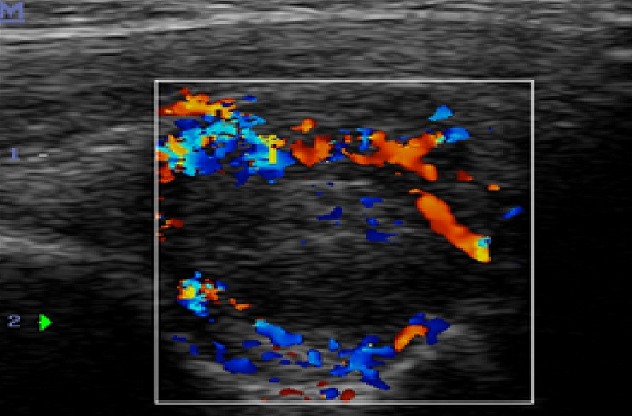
Enfant 08 ans, hémophile A modéré. Echographie conventionnelle en coupe sagittale de la face antérieure du coude gauche montrant un épaississement de la synoviale avec important flux vasculaire au codage Doppler et collection de liquide échogène intra-articulaire: hémarthrose avec synovite
